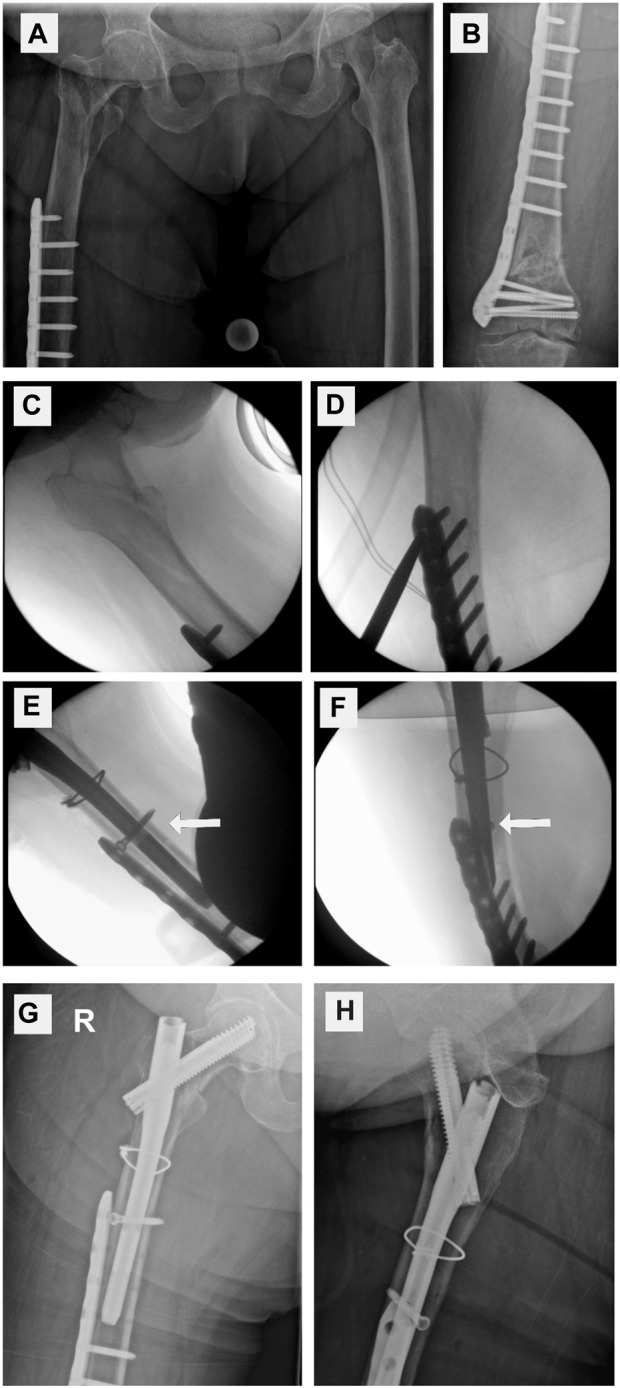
Clinical case without implant docking. (A,B) Proximal peri-implant femur fracture next to an existing distal femur plate (C–F) indicates the removal of several screws that keep the plate in place. The white arrow indicates a distal interlocking screw that is outside the plate, thereby showing no docking. (G–H) Postoperative X-ray of fracture fixation without nail–plate docking technique.
